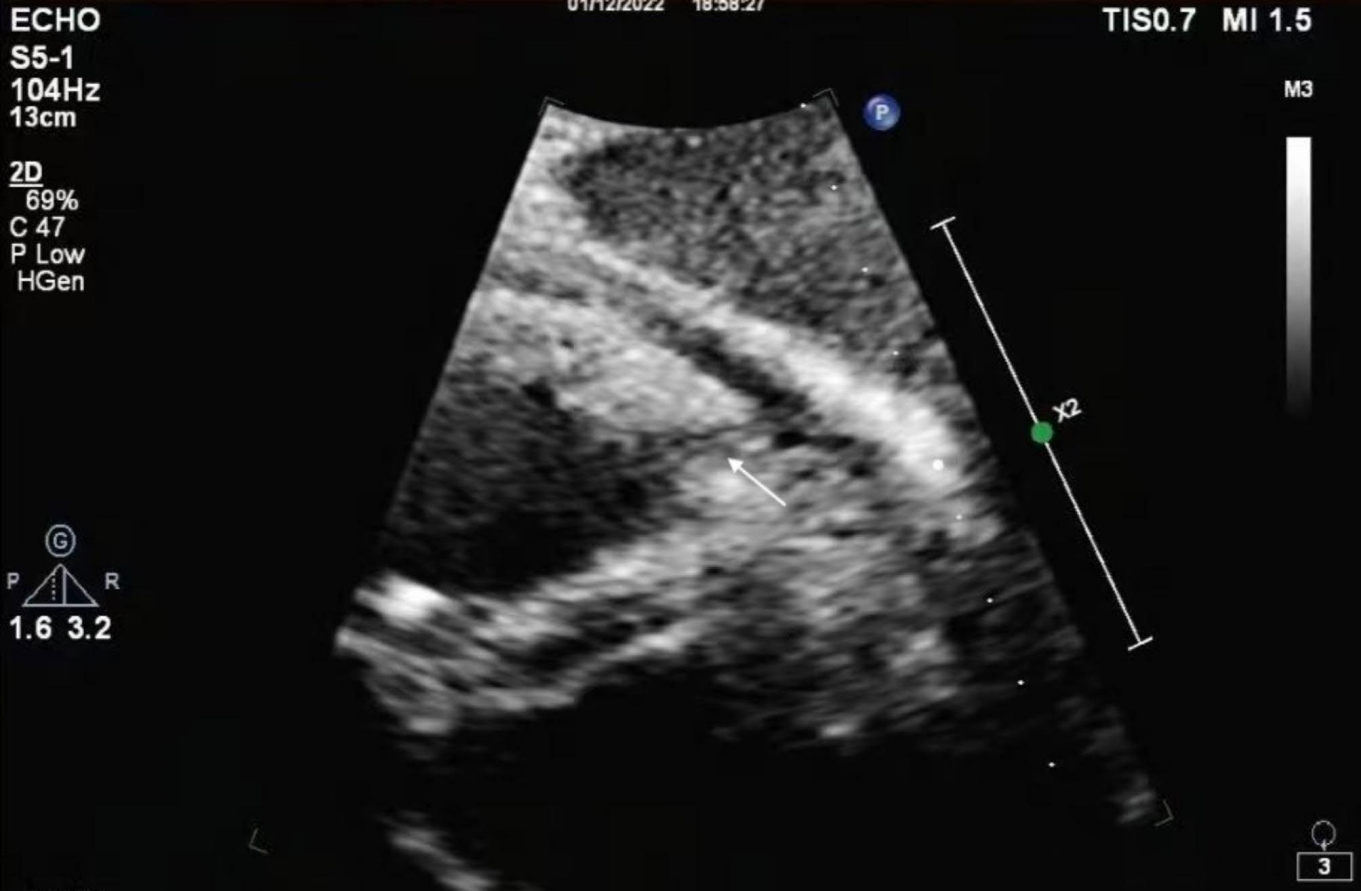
Postoperative cardiac echocardiography revealed an endocardial tear in the apical region of the right ventricle
